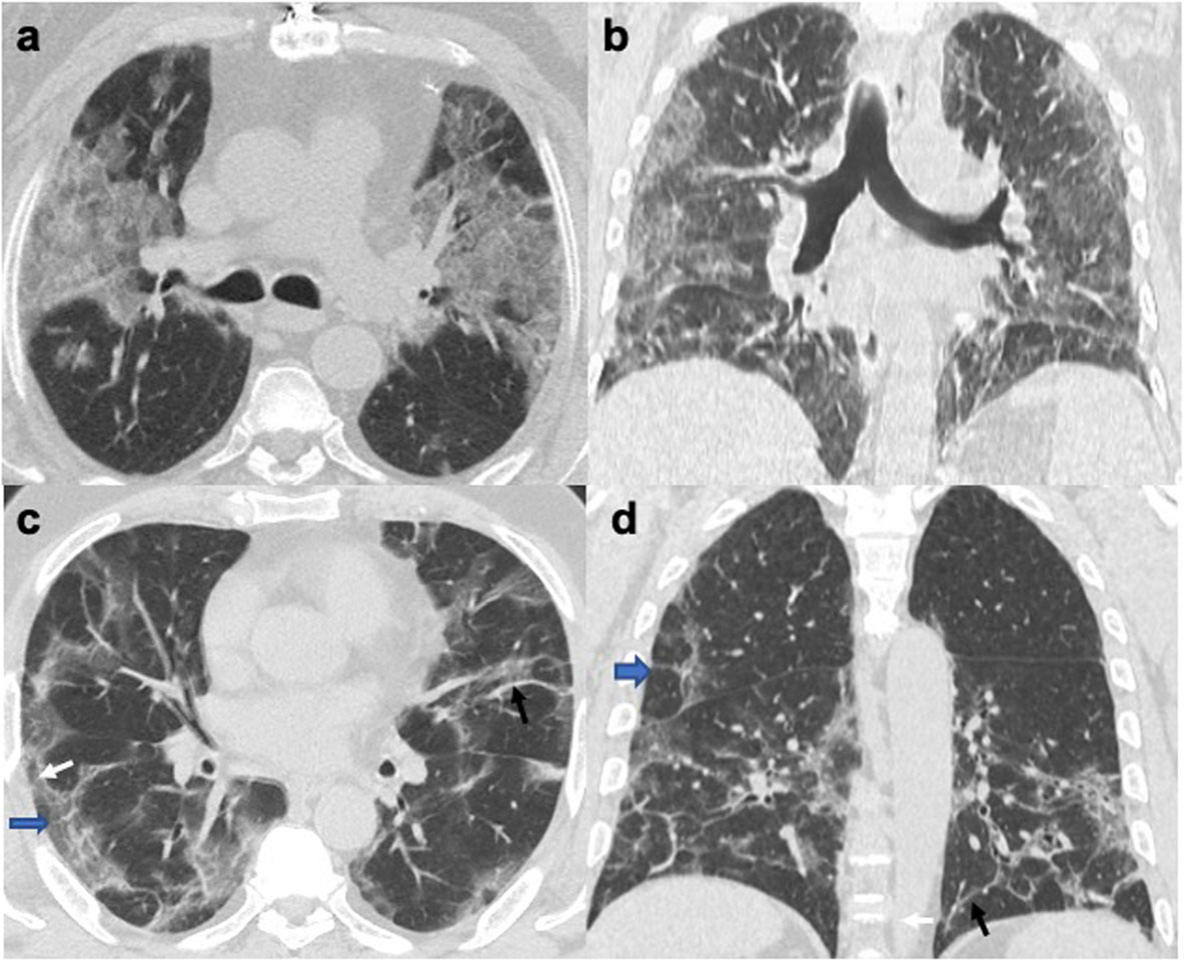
Axial (a, c) and coronal (b, d) CT slices of the lung window in different cases with COVID-19 pneumonia (a, b) showing the “crazy-paving sign” shown as bilateral ground-glass density with prominent interlobular and intralobular septations. c, d The bilateral parenchymal bands (black arrows), inter- and intralobular septations (blue arrows), and subpleural bands (white arrows), forming the spiderweb sign
